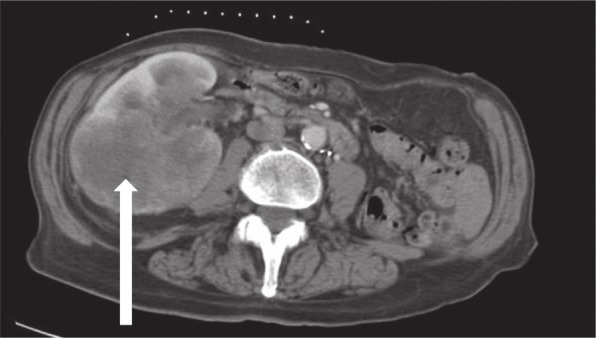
Axial computed tomography of the abdomen of patient 1 at the level of kidneys showing right renal metastases of primary thyroid sclerosing mucoepidermoid carcinoma with eosinophilia (arrow)
